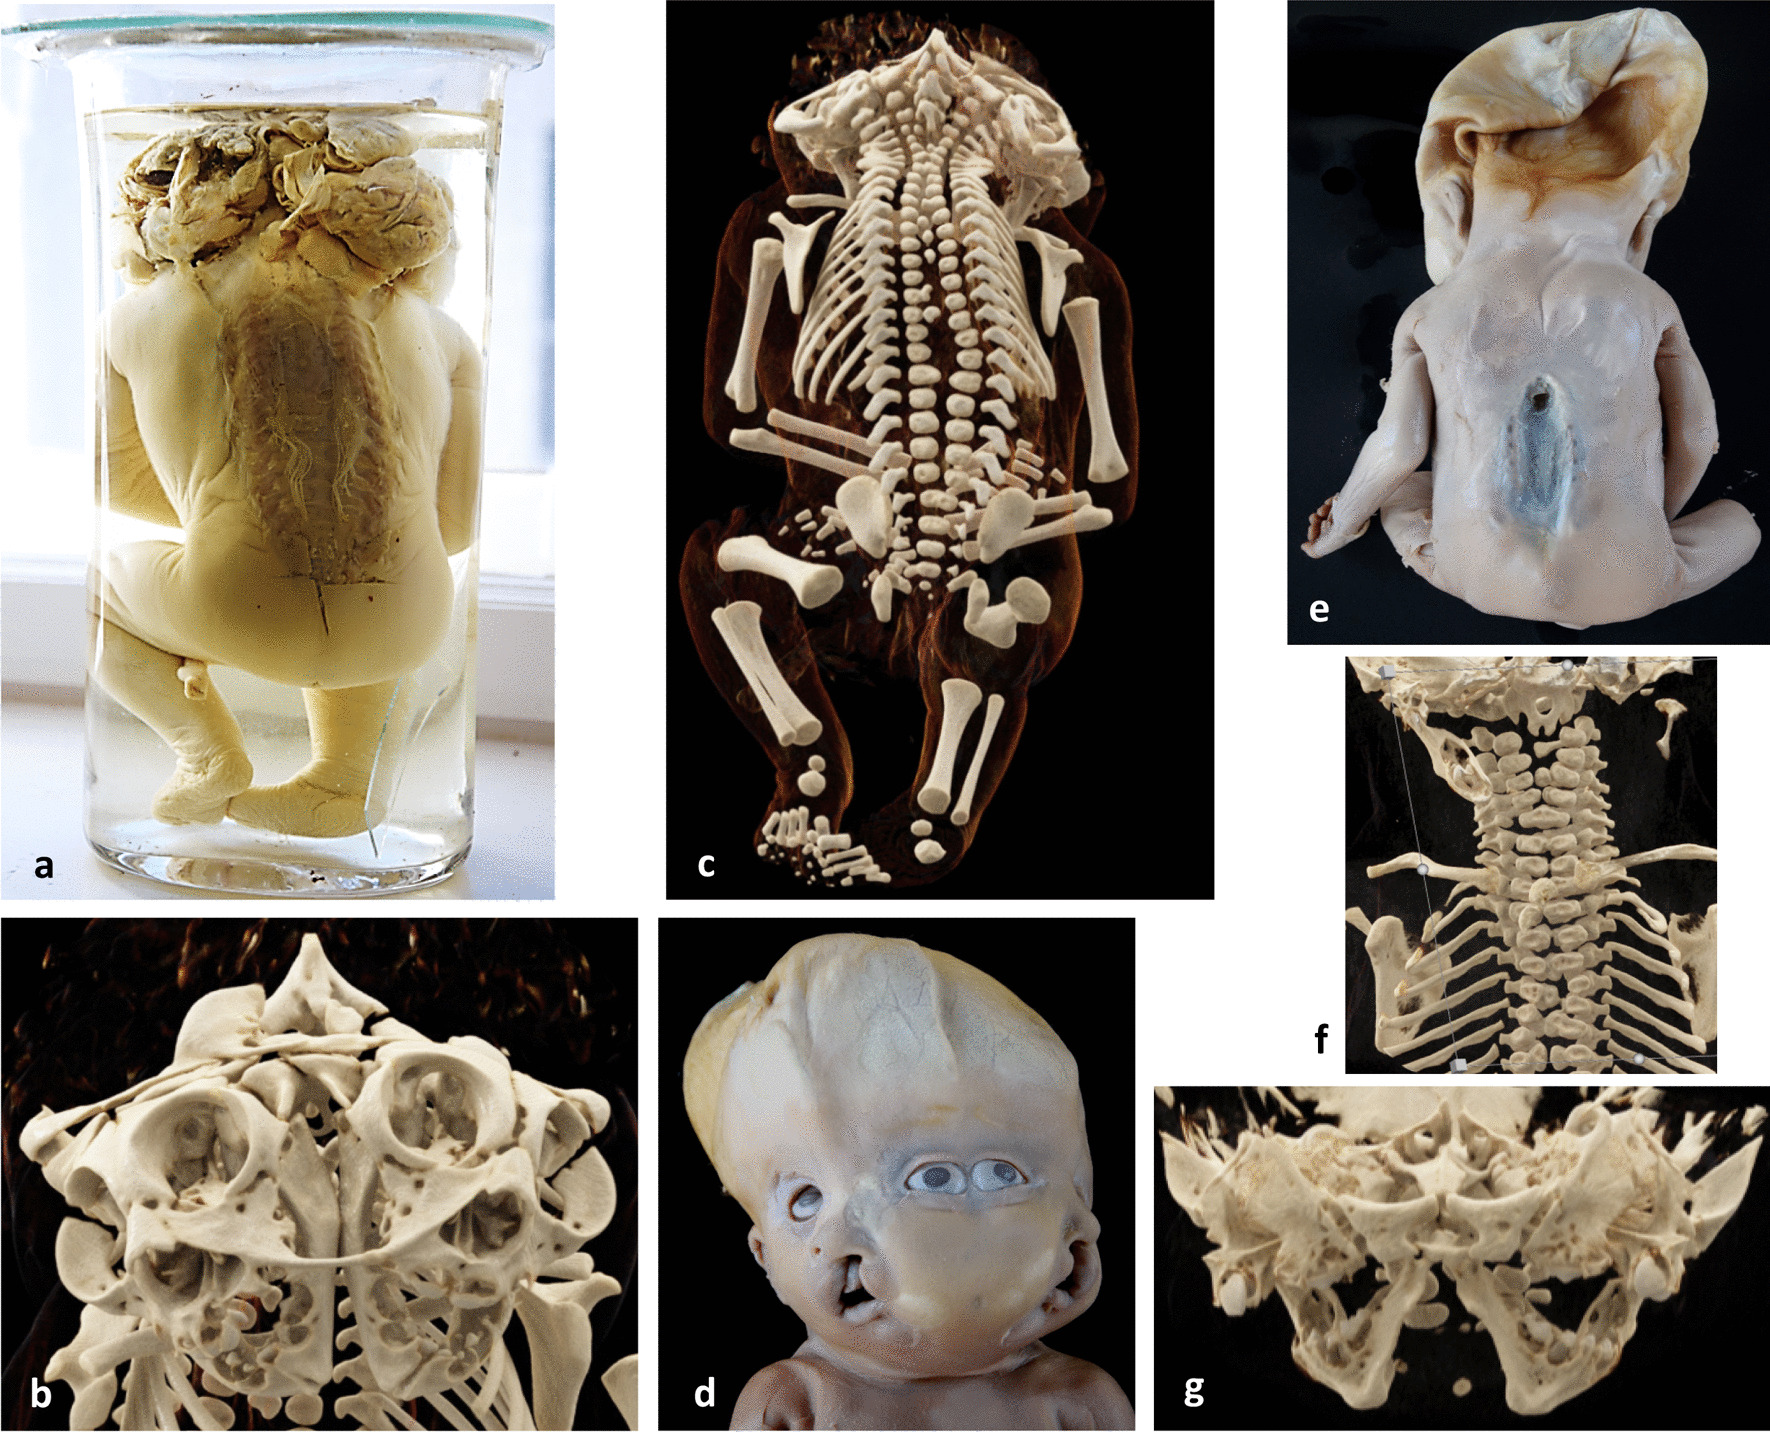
Case 4-Dorsal view of a female 3D-CT images of cases 4 and 5. Dorsal view of female diprosopus showing exencephaly and extensive craniorachischisis (a); frontal view of the faciial 3D-CT, showing acrania, osseous spike in between the duplicated skull base, duplication of facial bones, partial defects of median temporal bones and handle-shaped interfacial midline fusion of the median zygomatic processes (b); dorsal view of the fetal skeleton in 3D –CT displaying craniorachischisis, duplication of vertebral bodies down to L5 and splitting of the upper cervical spine (c). Case 5—Frontal view of the face of a male diprosopus terophthalmus with fused orbits, median ear pit and contralateral CLP (d); dorsal full length immage displaying collapse of the skull due to preexisting hydrocephaly and thoracolumbar rachischisis (e); frontal view of the upper spine in 3D-CT showing duplication of vertebral bodies, but not of vertebral arches and single foramen occipitale magnum (f); frontal view of facial 3D-CT presenting duplication of facial bones, defect of the inner temporal bones and narrowing, but no fusion of the zygomatic processes (g)
